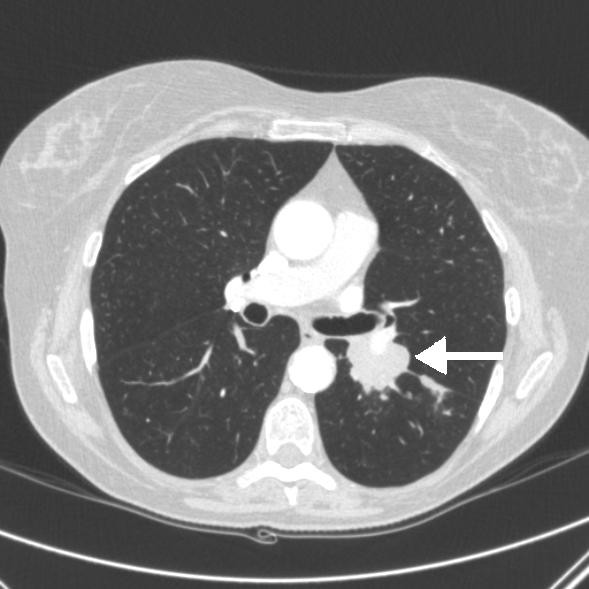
Computed tomography (CT) scan showing parenchymal opacity in the apical segment of the left lower lobe (arrow), highly suggestive of lung malignancy.
